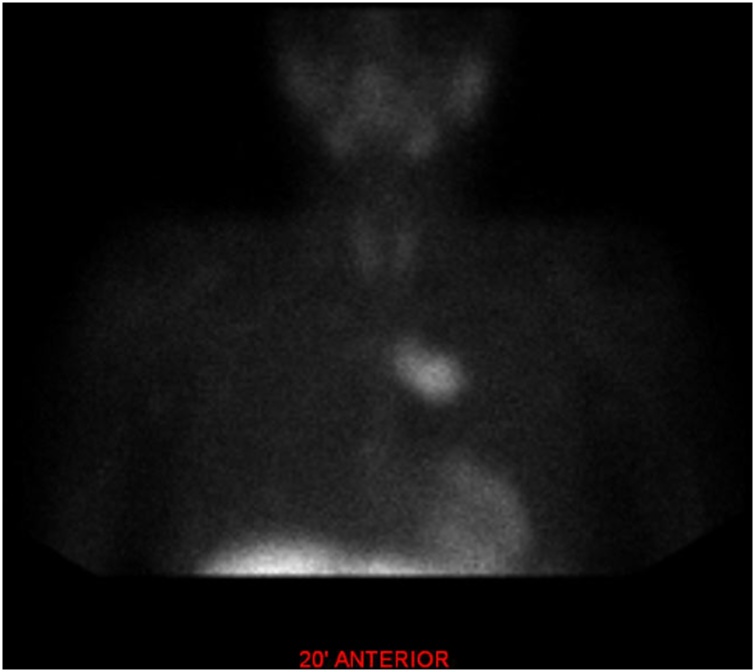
Tc-99m-MIBI scintigraphy detected an ectopic adenoma located in the lower anterior mediastinum, on the left of the median line.
